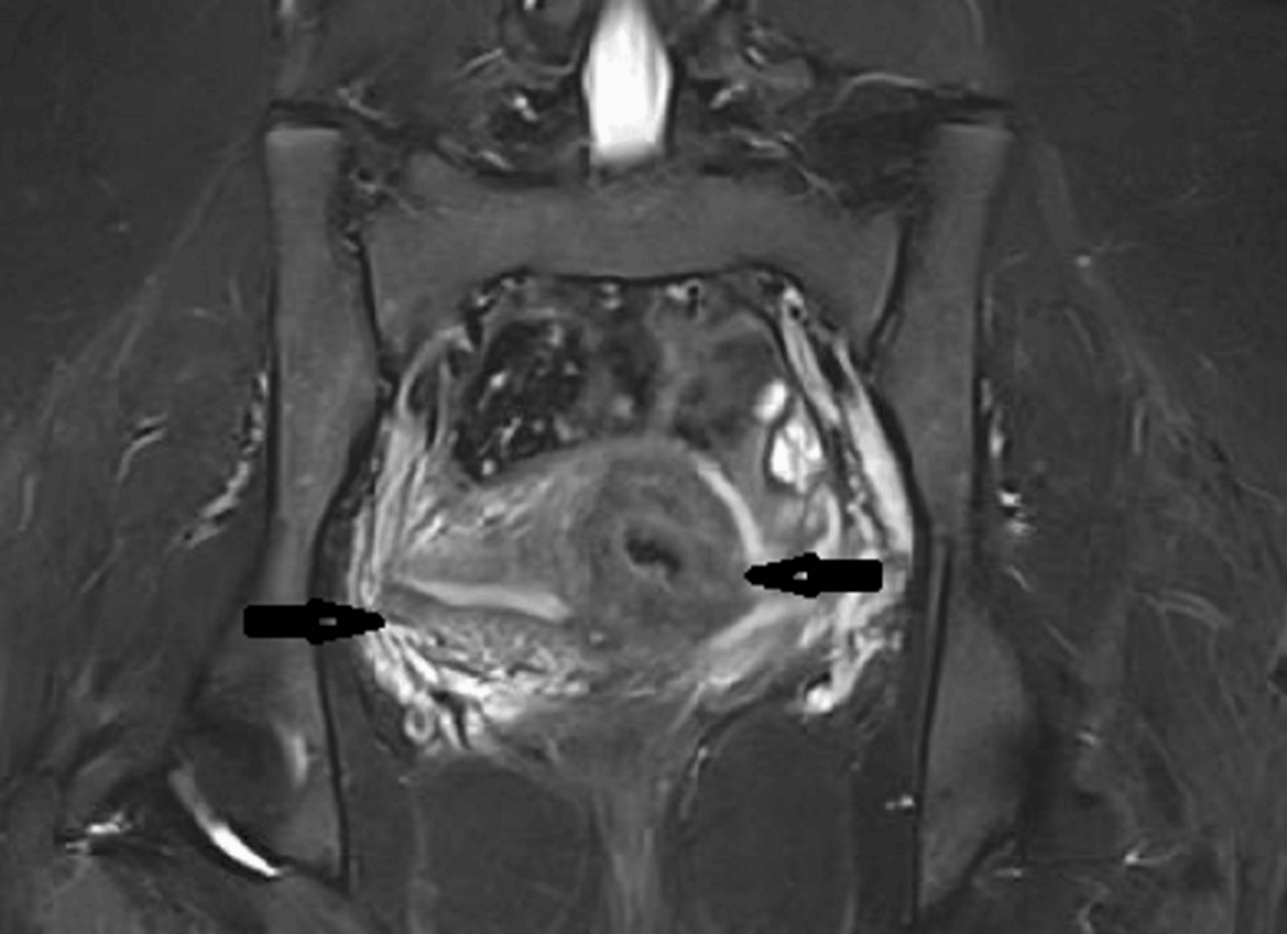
MRI showed a normal uterine cavity (arrow) with a distinct, round, noncommunicating cavitated lesion (arrow). A 3 cm x 4 cm mass is visible along the left anterior uterine wall, directly beneath the insertion of the round ligament. The T2 hyperintense endometrium lining the cavity contained hemorrhagic materials. On T1 images, it was hyperintense; on T2-weighted (T2W) images, it was hypointense.
